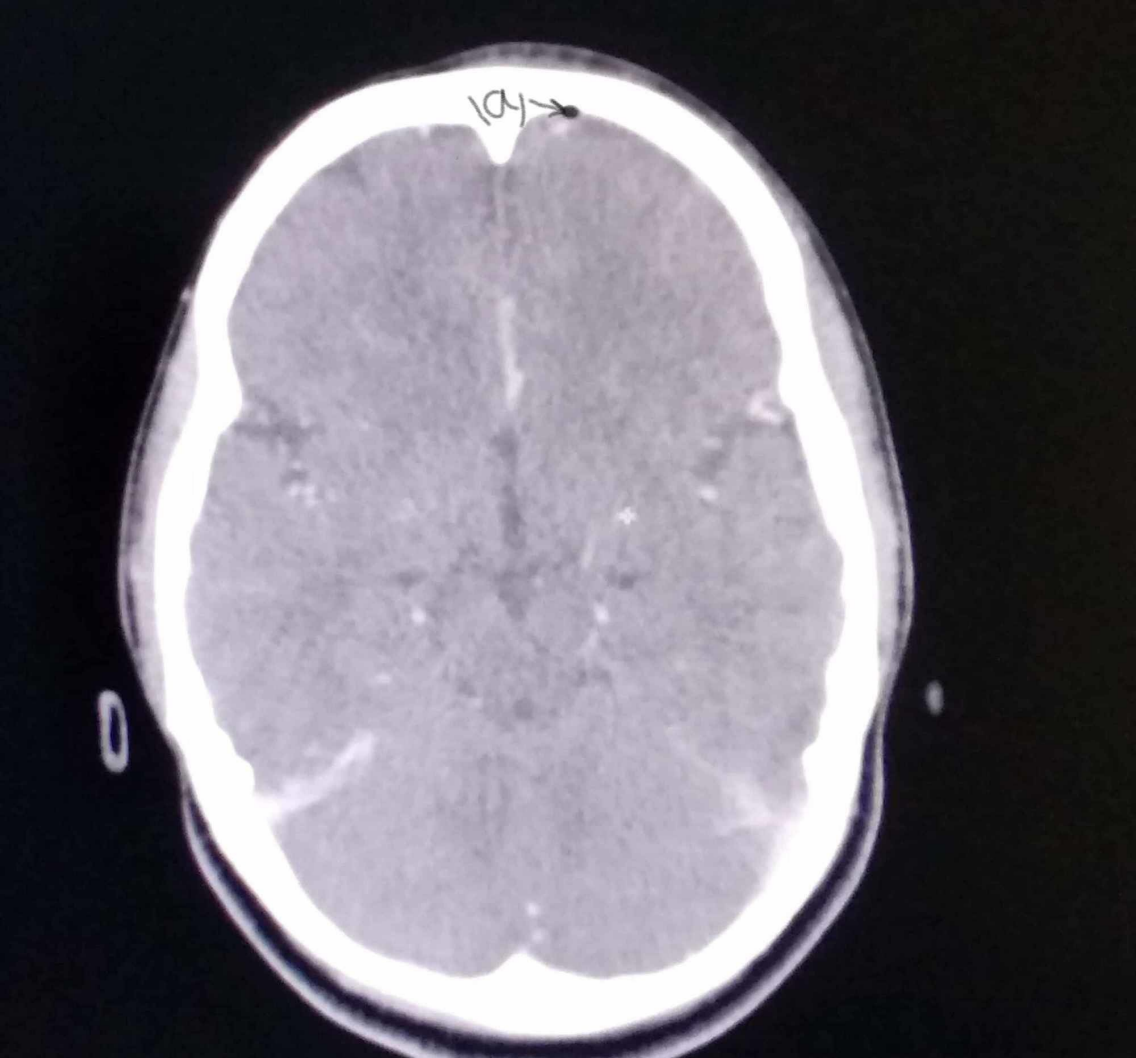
Axial CT scan, marked (a) is pneumocephalus in the frontal region
